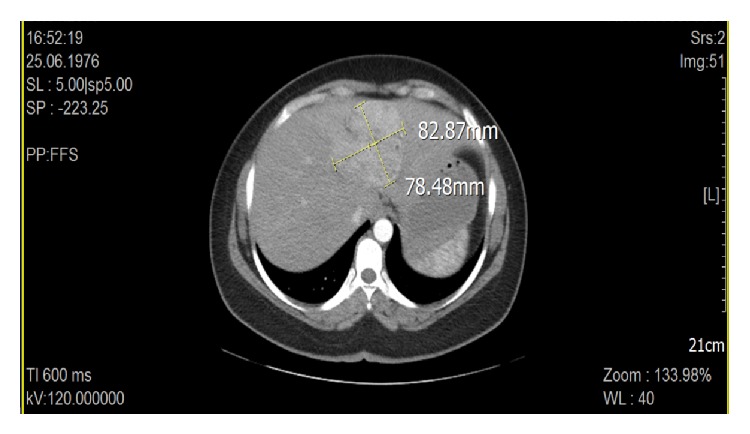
CT appearance of the tumor in the liver (CT image of hepatic mass).
